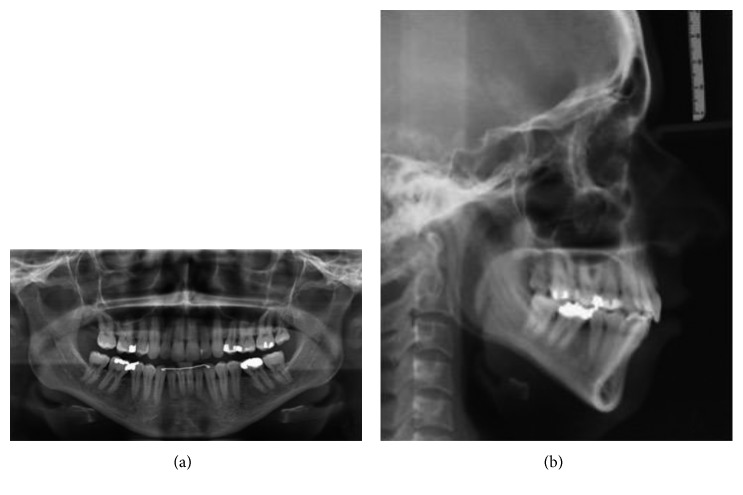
Six-year follow-up panoramic (a) and lateral (b) radiographs at 28 years, 8 months.
